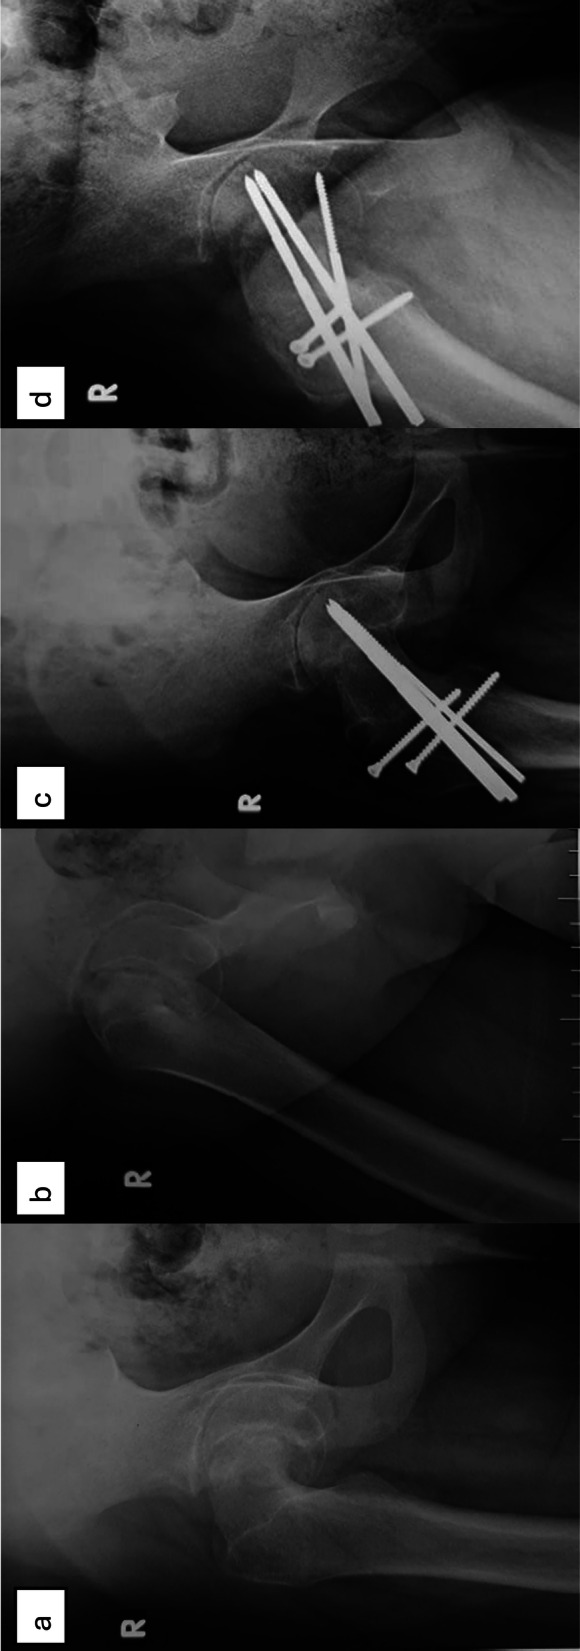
a, b Preoperative radiographs (ap and lateral) showing right SCFE in 11-year-old girl. c, d Postoperative radiographs (ap and lateral) at final follow-up visit (7 years) and after fixation by three Schanz screws (3 mm)
